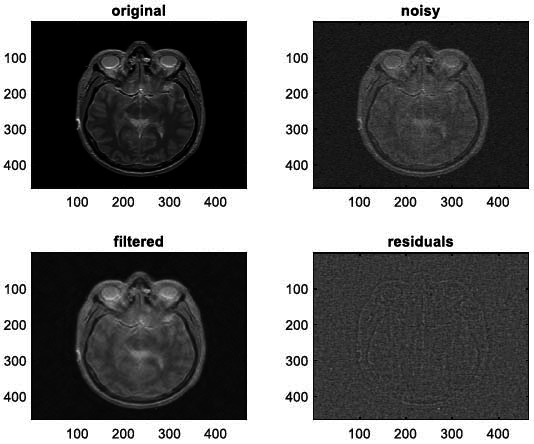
The eighth example of MRI denoising by applying the proposed image denoising technique.
